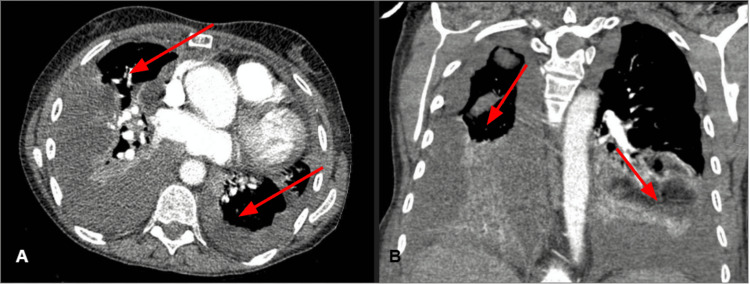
CT scan obtained following abrupt hypoxic episode. Transverse (A) and coronal (B) view of CT chest revealing moderate bilateral pleural effusions (marked by red arrows) CT: computed tomography
